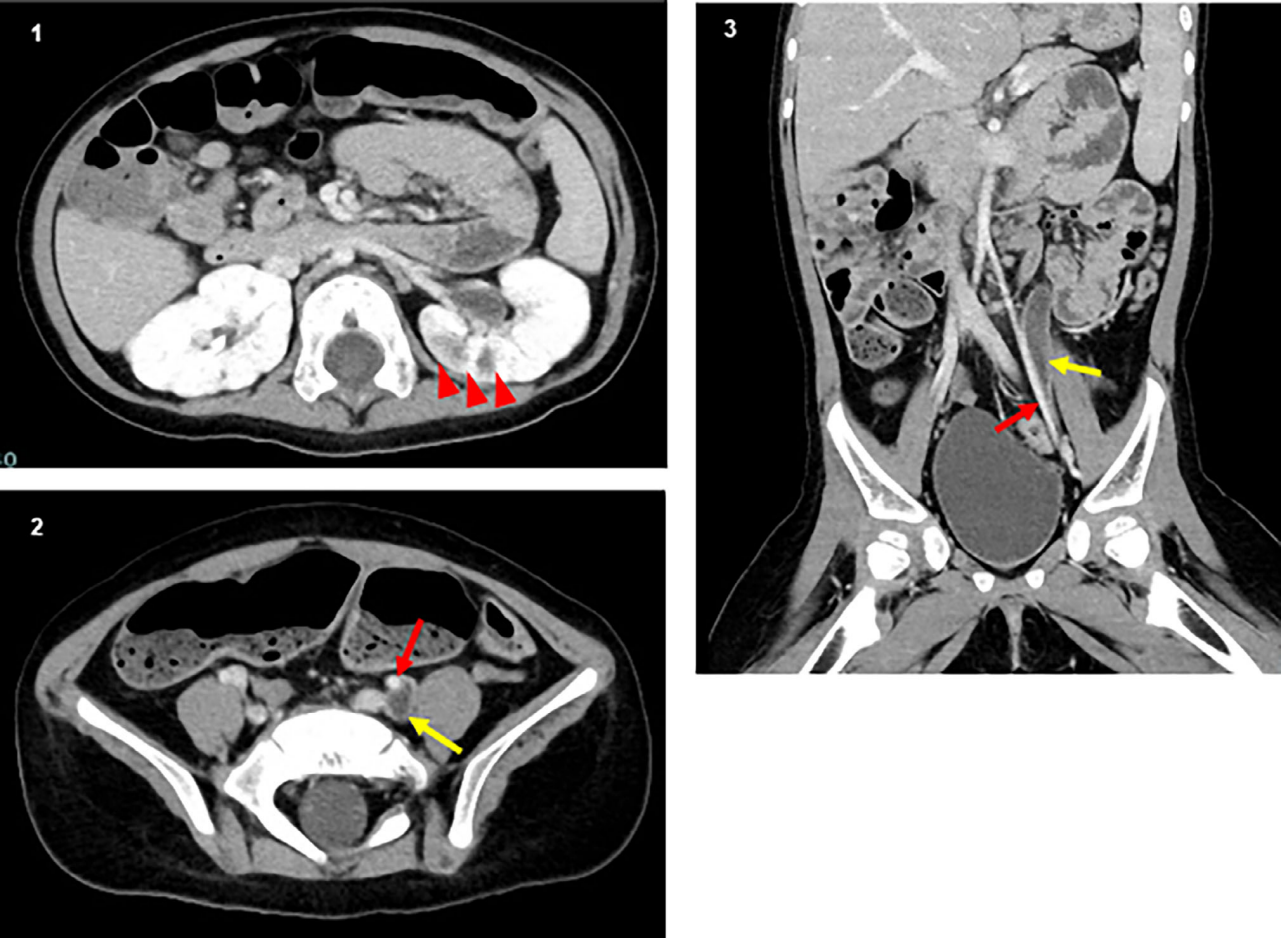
(1) Contrast‐enhanced CT showing acute focal bacterial nephritis in the left kidney (red triangles). (2 and 3) Contrast‐enhanced CT showing the retroiliac ureter. Left ureter courses posterior to the left iliac artery. Yellow arrow: hydroureter; red arrow: left iliac artery.
